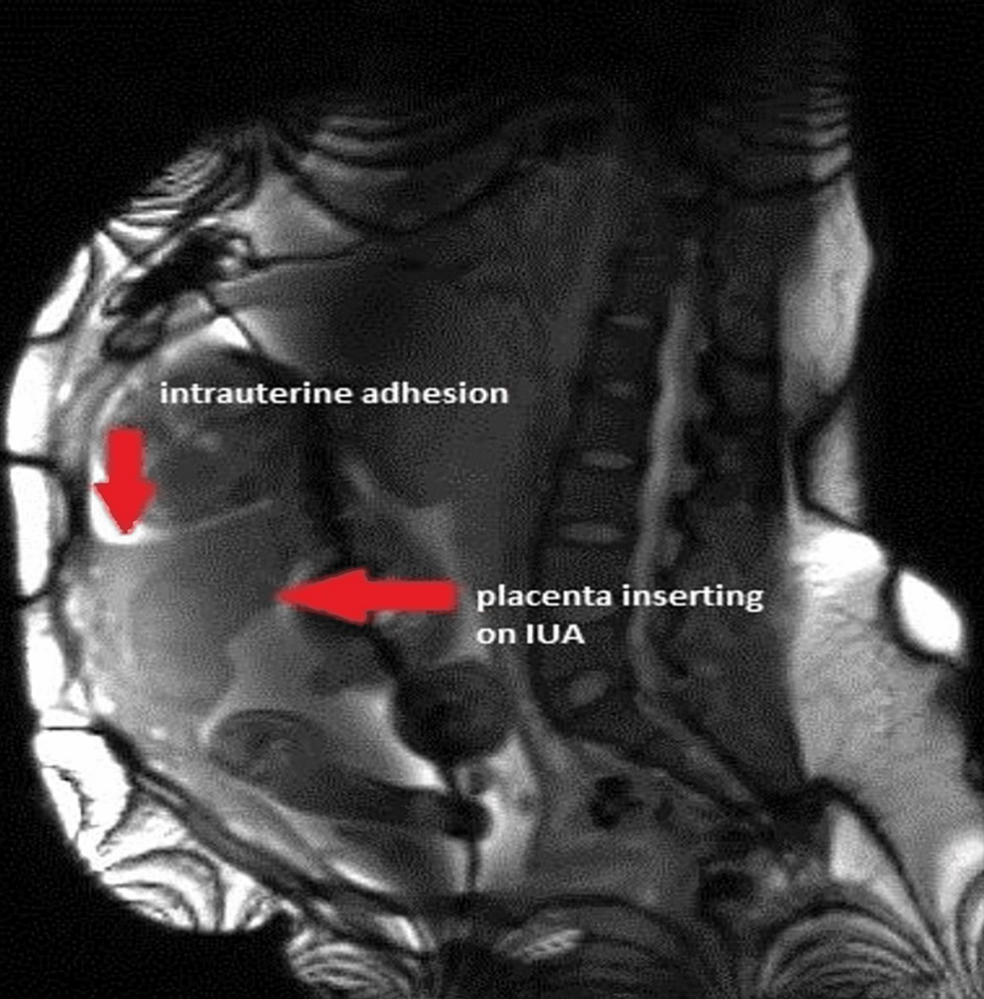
Sagittal MRI displaying an antero-posterior adhesion (vertical red arrow) and placental insertion covering two-thirds of the adhesion (horizontal red arrow). MRI, magnetic resonance imaging
